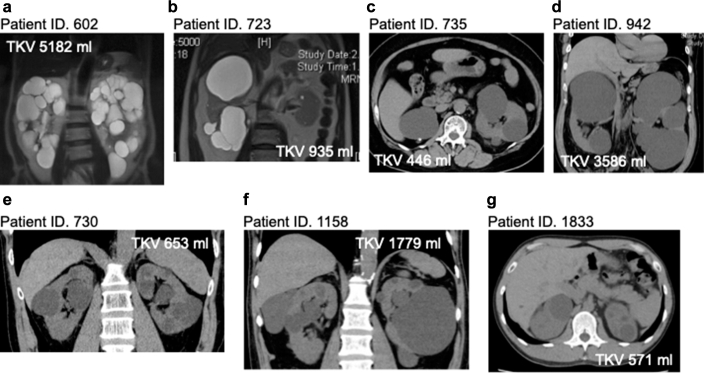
Computed tomography or magnetic resonance images of the kidneys in patients with pathogenic variants in *IFT140*. The TKV varied among these patients. Regarding kidney cysts, each cyst was larger than typical ADPKD, with some cases being asymmetrical. (a) Patient ID.602. (b) Patient ID. 723. (c) Patient ID.735. (d) Patient ID. 942. (e) Patient ID.730. (f) Patient ID. 1158. (g) Patient ID. 1833. ADPKD, autosomal dominant polycystic kidney disease; TKV, total kidney volume.
